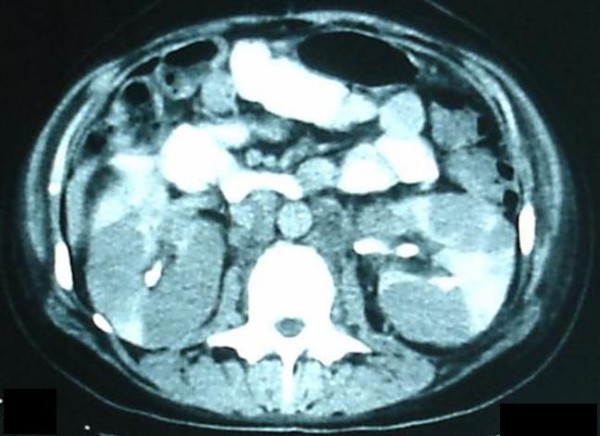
CT scan of the abdomen showing enlarged retroperitoneal lymph nodes and infiltration of both kidneys with hypodense masses compatible with lymphomatous involvement.
